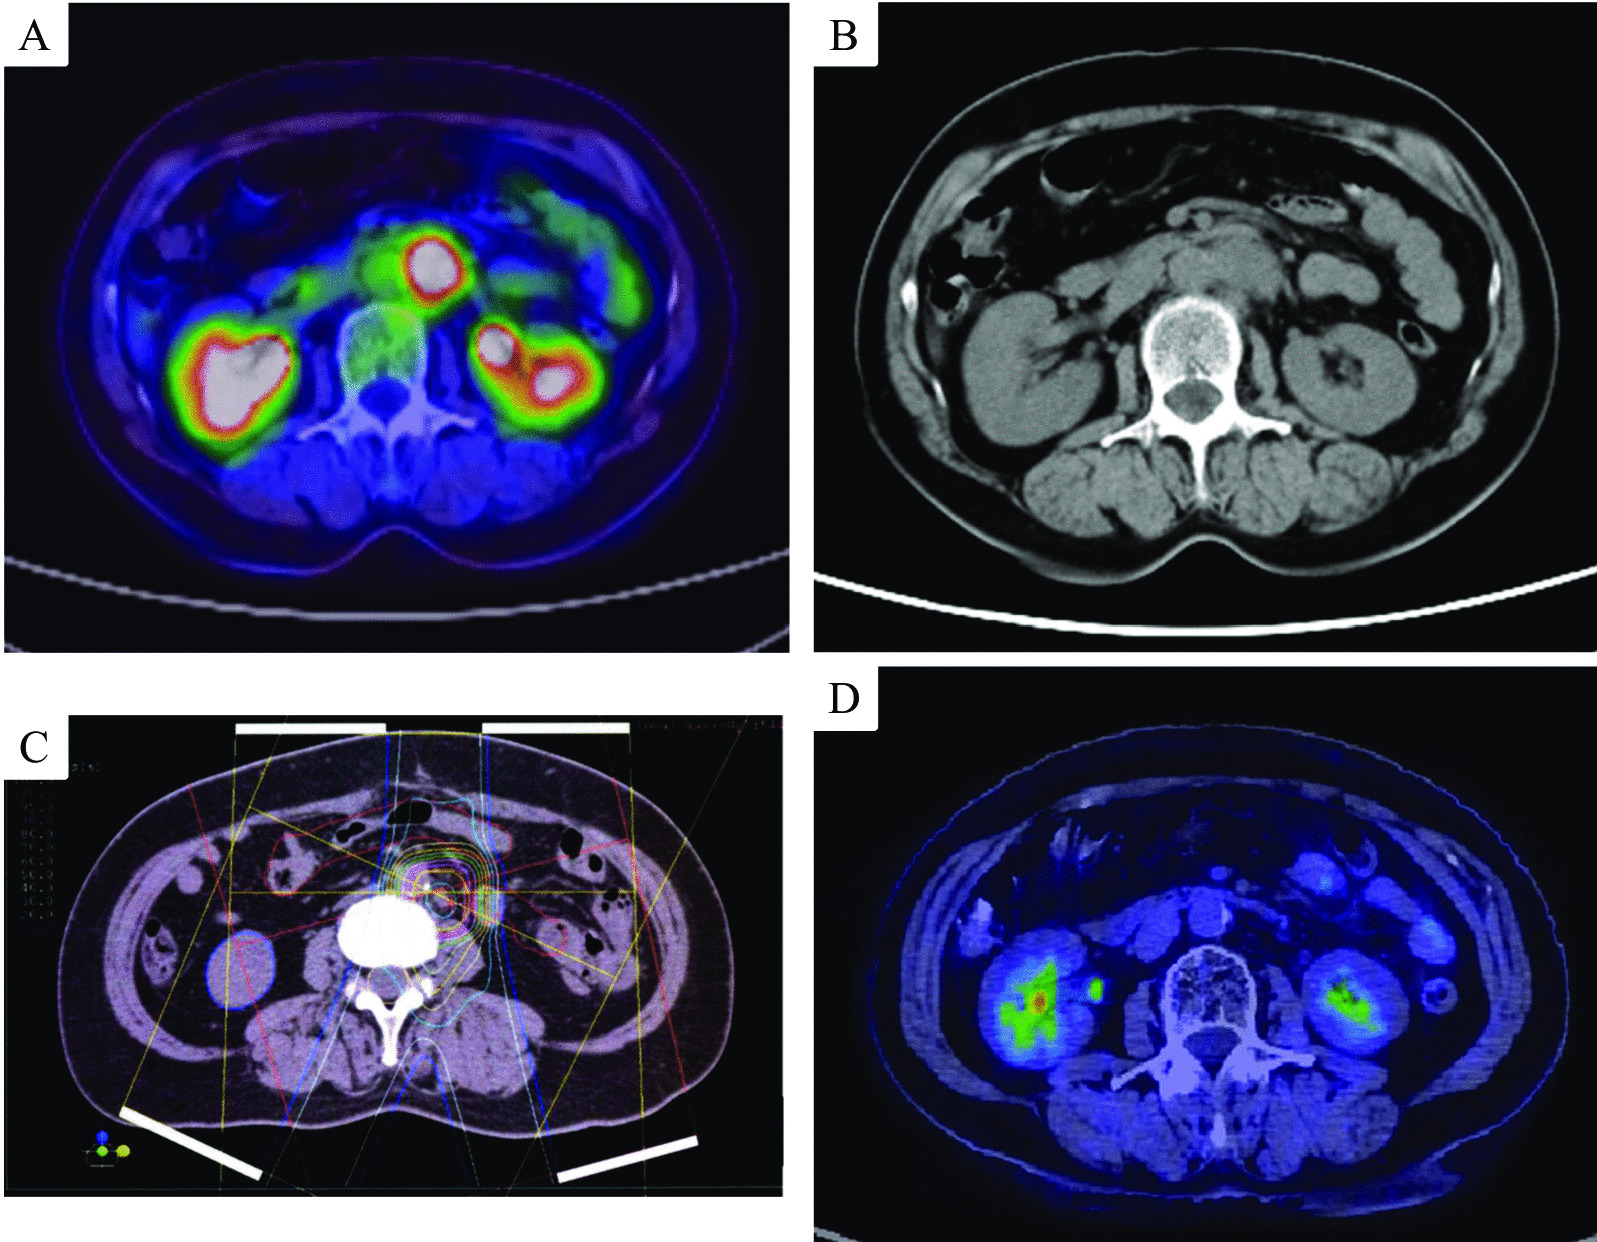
Positron emission tomography/computed tomography (PET/CT) scans before proton beam therapy (PBT) (A). A solid tumor with the abnormal accumulation of 2-deoxy-2-(18F)fluoro-d-glucose (SUVmax = 19.44) was observed adjacent to the left renal vein (B). A dose distribution curve of the axial and sagittal sections C orange, purple, pink, green, yellow, sky blue, and dark blue lines in the circle represent doses of 5225, 4950, 4400, 3850, 2250, 1650, and 550 cGyE, respectively, which were delivered to the patient over the course of the PBT regimen. PET/CT scans of the para-aortic lesion after PBT (D). The recurrent para-aortic lesion was completely eradicated
